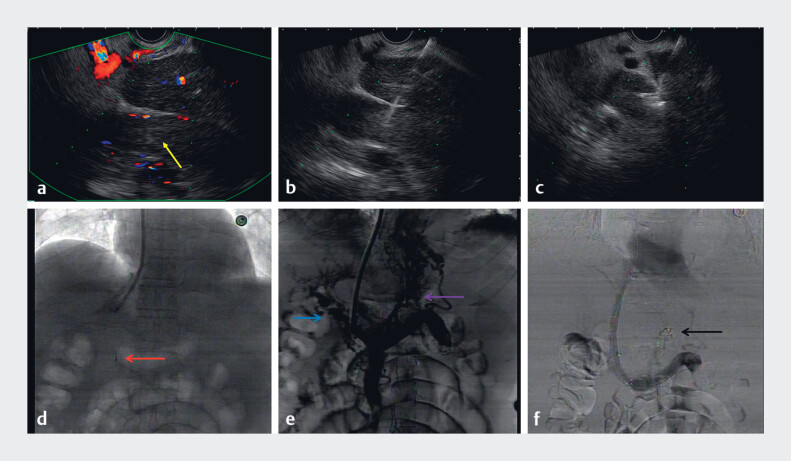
Endoscopic ultrasound (EUS) and radiography images.
